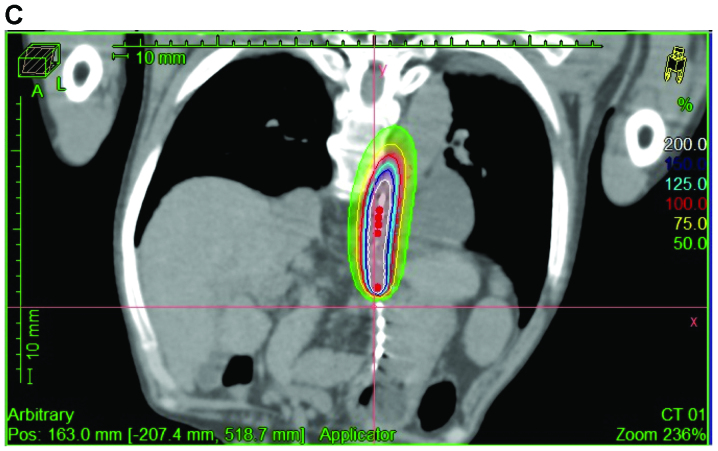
Intracavitary radiation therapy dose distribution in the (A) axial, (B) sagittal and (C) coronal plane using planning computed tomography.
